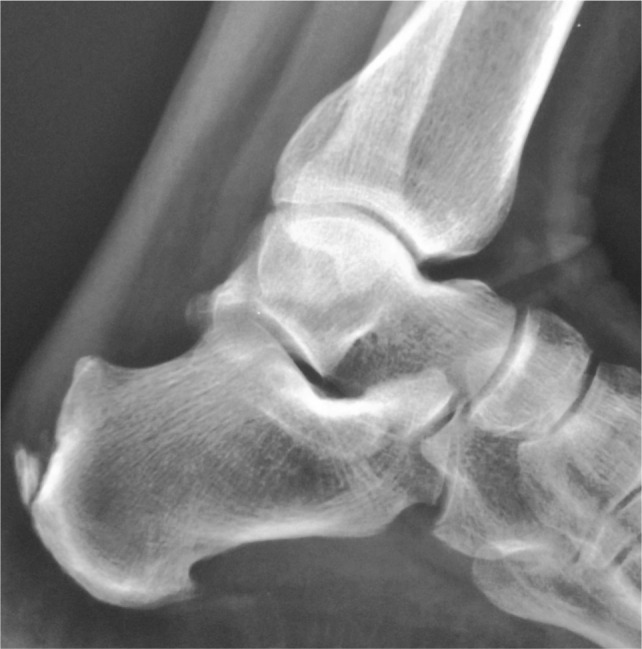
Preoperative radiograph Showing Haglund Deformity.
